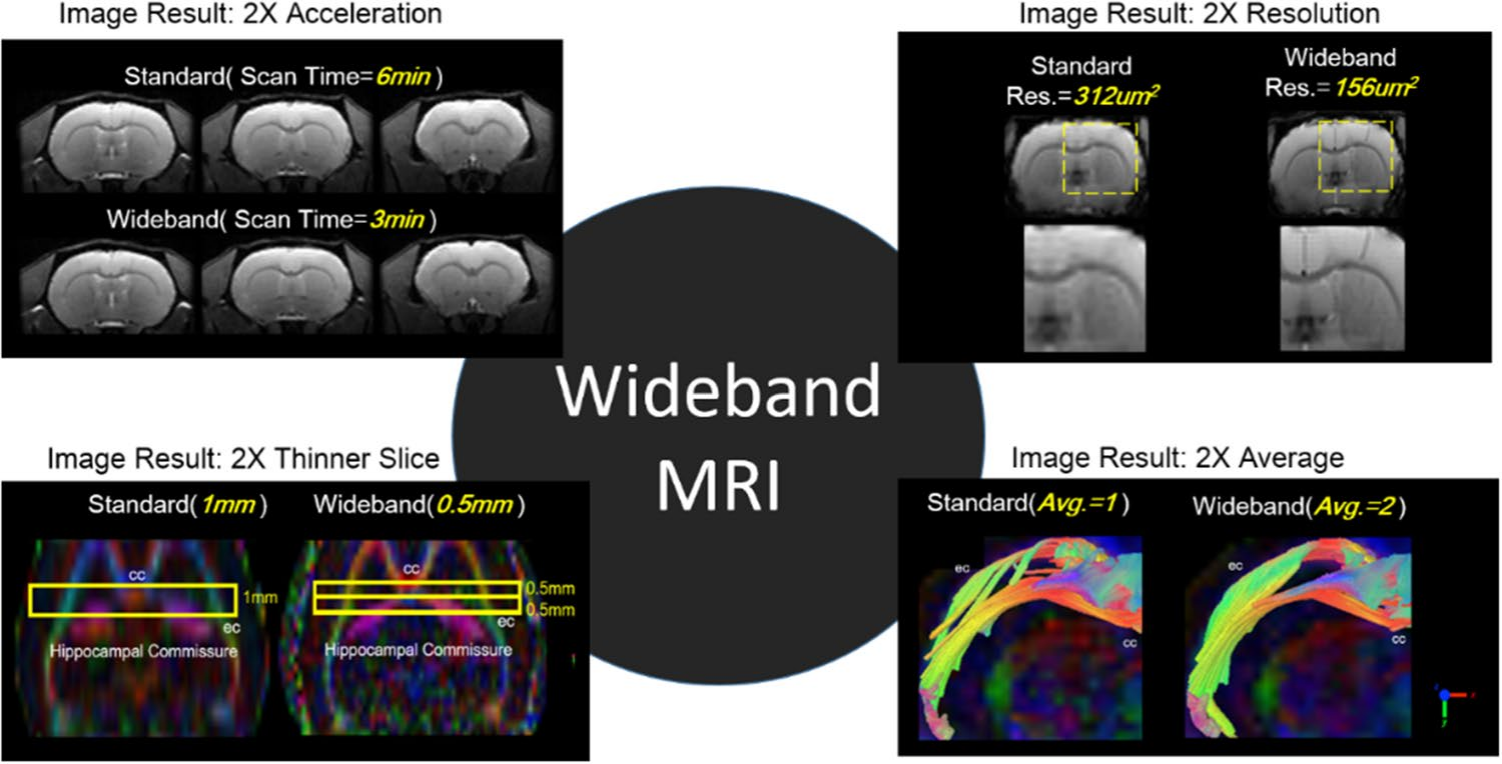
Multiple applications of the Wideband MRI. This study demonstrated multiple benefits of the Coherent Wideband EPI technique, including temporal/spatial resolution enhancement, SNR improvement, and accuracy study.
